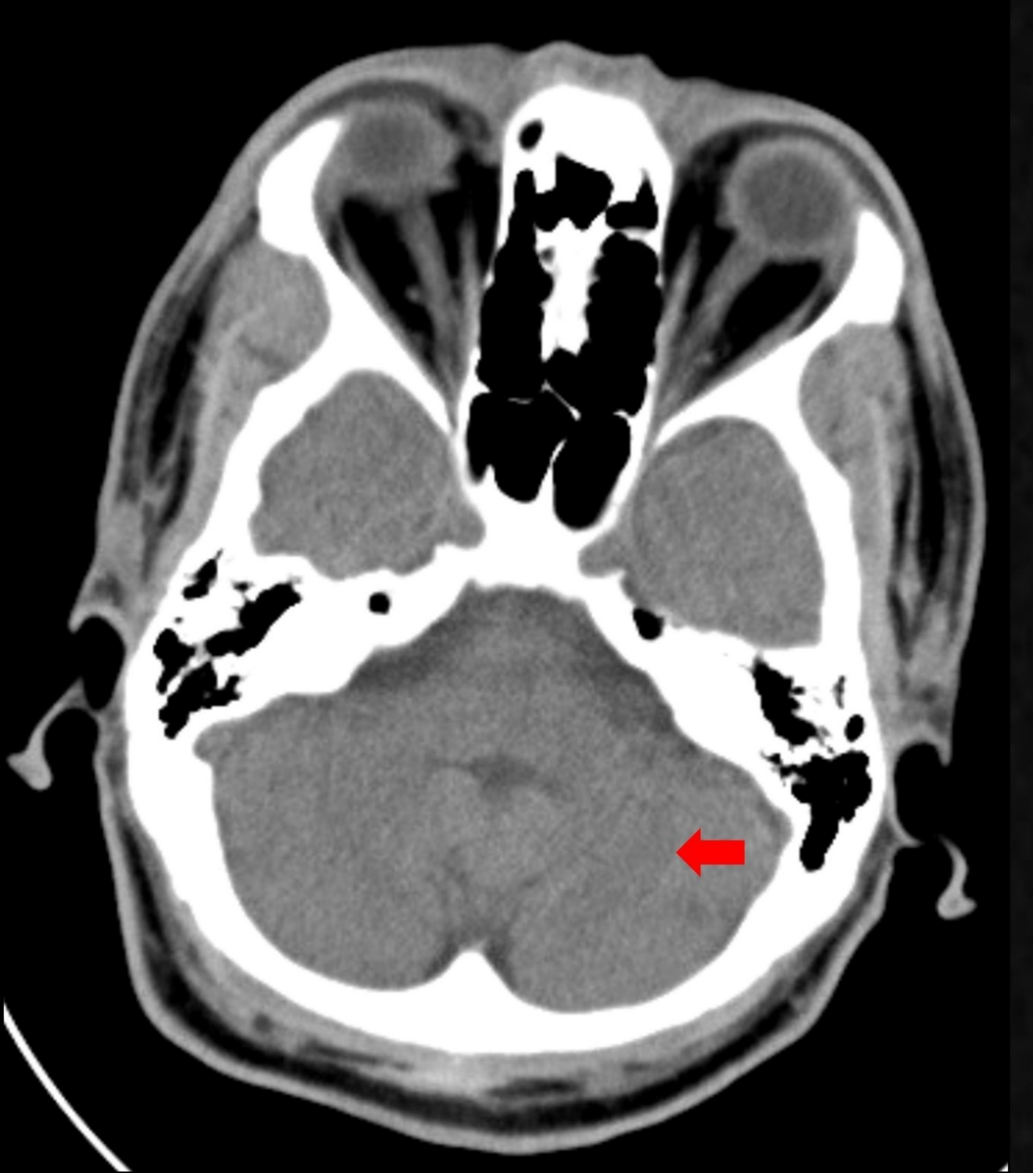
Noncontrast head computed tomography scan. Computed tomography of the head showed subtle focal area of hypoattenuation (red arrow) in the left cerebellar hemisphere.
